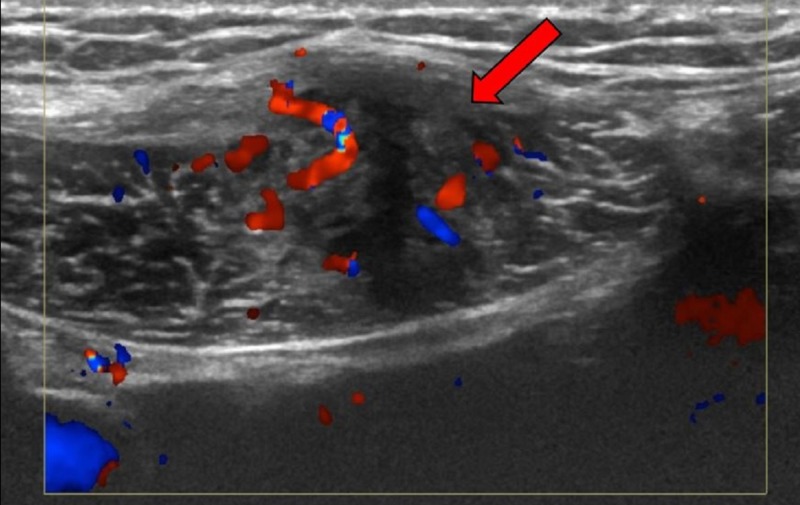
Pelvic ultrasound Ultrasound of the right lower quadrant anterior abdominal wall demonstrates a complex intramuscular mass with internal vascularity (as evidenced by the red and blue Doppler signal) in the lower right rectus abdominis muscle
